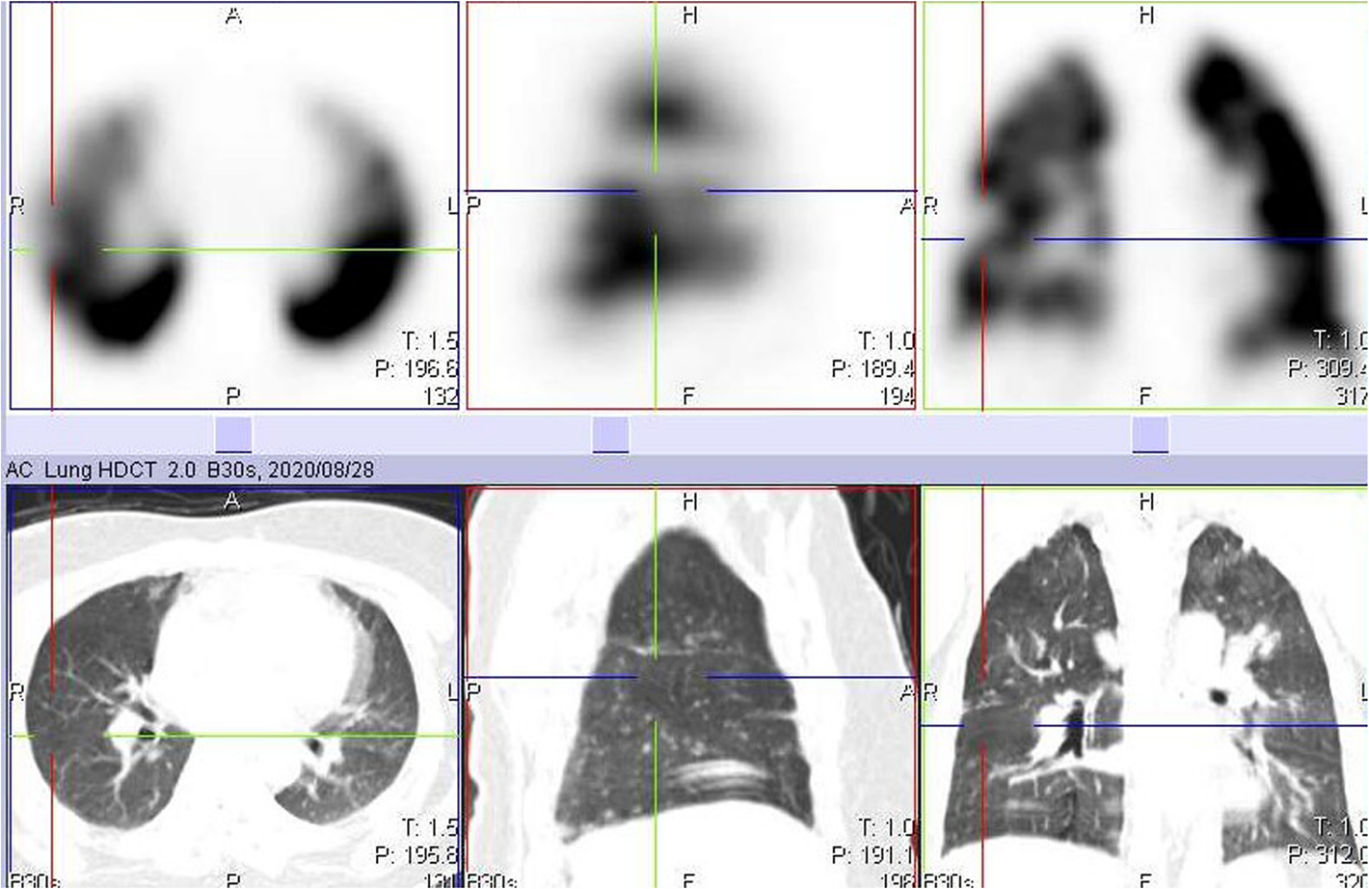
Axial, coronal and sagittal SPECT images of the lungs showing a large perfusion defect in the lateral segment of the right middle lobe. The corresponding CT images below do not show obvious structural lung changes in that segment
